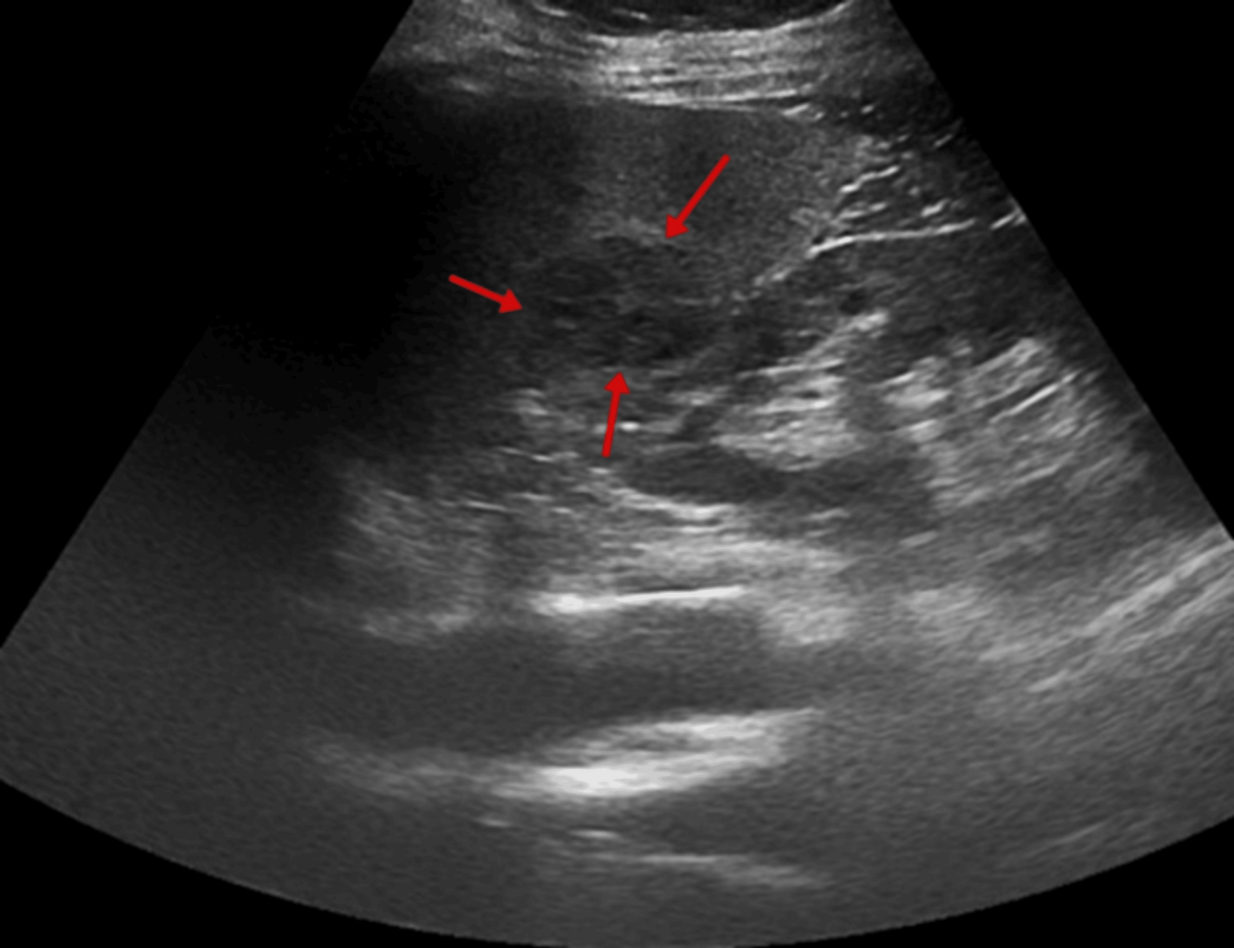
Gray-scale axial abdominal ultrasonography examination At the level of the splenic hilum, a nodular lesion measuring approximately 26x29 mm with mildly lobulated contours is observed. It appears iso-hypoechoic compared to the surrounding splenic parenchyma (red arrows).
